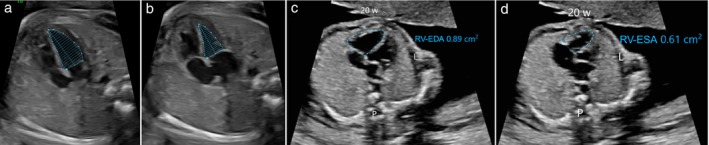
Assessment of ventricular ejection fraction (EF) and fractional area change (FAC). (a) EF is measured from a high frame rate (> 60 fps) apical four‐chamber view, avoiding ventricular foreshortening. Ventricular cavity measurement using Phillips Intelli Space Cardiovascular software (Phillips Healthcare, Andover, MA, USA) to calculate end‐diastolic volume (EDV) by tracing the ventricular endocardial border at end‐diastole (largest cavity, just before atrioventricular (AV) valve closure) is shown. (b) For measurement of end‐systolic volume (ESV), the frame with the smallest ventricular cavity, just before AV valve opening, is selected and the endocardial border traced from one side to the other of the AV valve annulus. EF is then calculated using the equation (EDV − ESV)/EDV. (c) Measurement of the right ventricular (RV) end‐diastolic area (EDA) using the image with the largest RV cavity is shown. (d) To obtain the RV end‐systolic area (ESA), RV is measured when the cavity is smallest. FAC (%) is then calculated as ((RV‐EDA − RV‐ESA)/RV‐EDA) × 100. For the 20‐week (w) fetus shown in (c,d), the FAC would be ((0.89−0.61)/0.89) × 100 = 31%. L, left; P, posterior.
